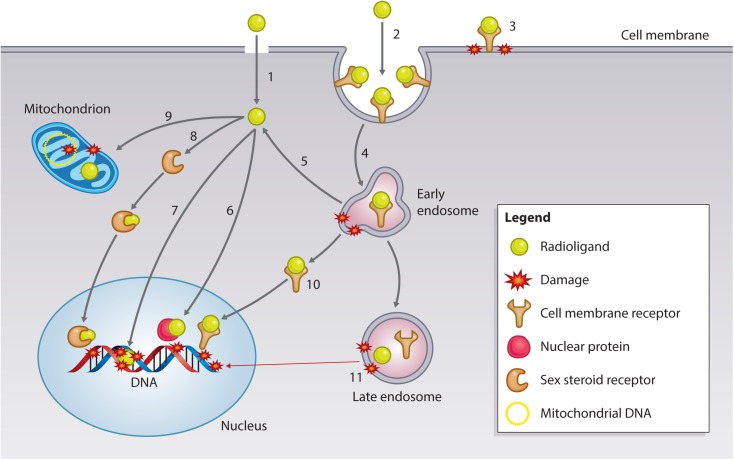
Subcellular targets of Auger electron-emitting theranostic radionuclides. Strategies to reach intracellular targets can broadly be categorized into radioligands that diffuse through the cell membrane by passive/active transport (1) or bind to cell membrane receptors. Membrane receptor-radioligand complexes can be internalized via endocytic pathways (2) or remain surface-bound (3), damaging the cell membrane via hydroxyl radical formation (see text for further explanation). Endocytosed radioligand-receptor can continue to damage endosomes (4), and certain radioligands have the potential to escape endosomal entrapment (5). Cytosolic radioligands can have various fates and targets. Approaches have utilized radioligands that can bind nuclear proteins, such as γH2AX or telomerase (6), or that can directly interact with the DNA (7). Some radioligand strategies involve targeting SSR (8), which can traffic to the nucleus of the cancer cell to exert damage. A more recently explored fate is mitochondrial targeting (9), which can lead to mitochondrial DNA damage and the generation of oxidative stress, resulting in mitochondrial-induced apoptosis. Endosomal escape can also occur for receptor-radioligand complexes, which can travel to the nucleus, as has been found with targeting of the EGFR family (10). Most complexes are unable to escape the endosome and will be sorted out of the cell via large endosomal/lysosomal vesicles. While being processed, radionuclides can continue to do damage endosomal vesicles, and irradiate genomic DNA in case of long track-path radionuclides emitters, such as ^177^Lu-/^225^Ac-PSMA or ^177^Lu-DOTATATE) (11).
